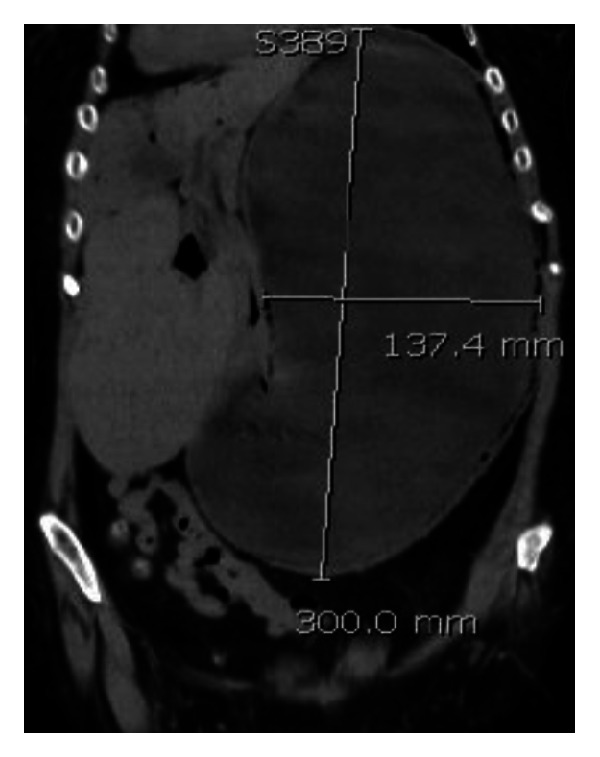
Gastric dilatation on CT scan, coronal reformat.
